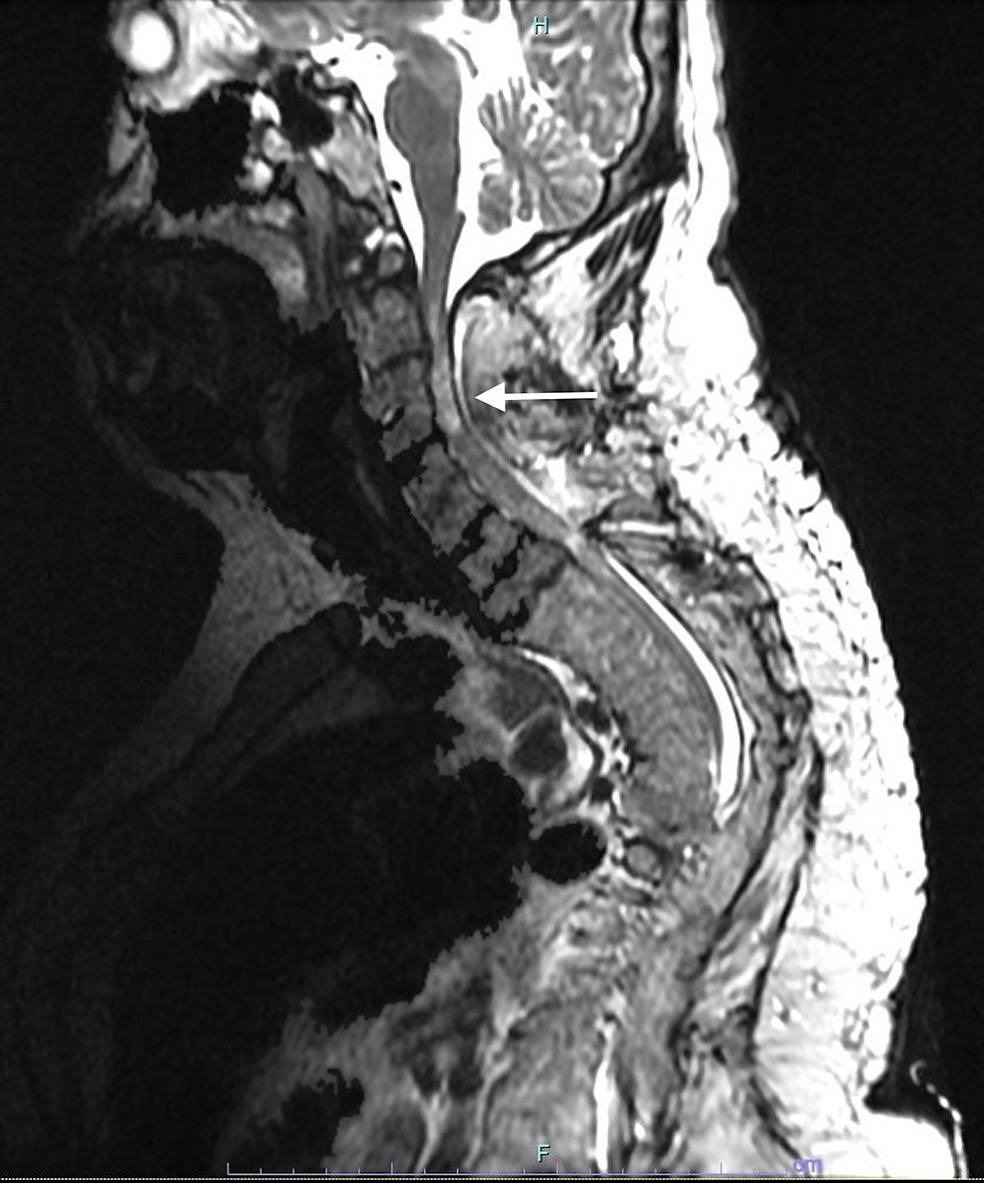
Postoperative MRI. Postoperative MRI of the cervical spine revealed decompressive laminectomy with substantial C2-C5 cord edema (indicated by the arrow) without pathological diffusion restriction to suggest irreversible ischemia.
